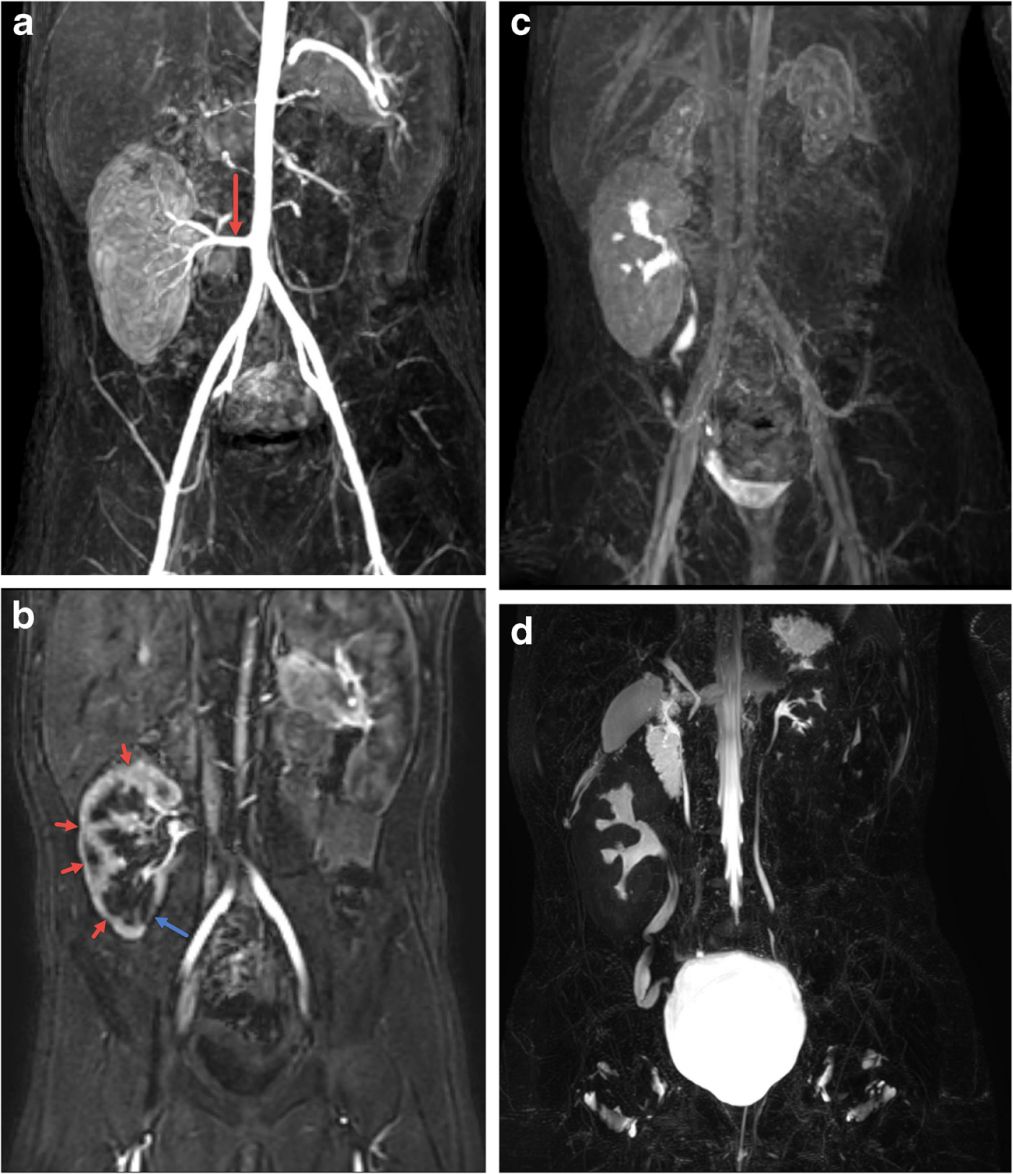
Nine-year-old with history of hemolytic uremic syndrome who has undergone right lower quadrant renal transplant. a Maximum intensity projection (MIP) reformat image from the arterial phase of the dynamic post-contrast sequence shows a patent artery supplying the right lower quadrant renal transplant (arrow). b Subsequent MIP image from the corticomedullary phase shows multifocal regions of parenchymal thinning and scarring (red arrows) with the most pronounced region of scarring in the lower pole medially (blue arrow). c MIP delayed post-contrast excretory phase image shows excretion of contrast into the renal transplant collecting system and ureter without dilation and with free passage of contrast into the bladder. The small native kidneys can also be seen. d MIP reformat of 3D T2-weighted fast spin-echo image shows no pelvocaliectasis with mild tortuosity of the ureter. The small native kidneys and ureters can also be seen
